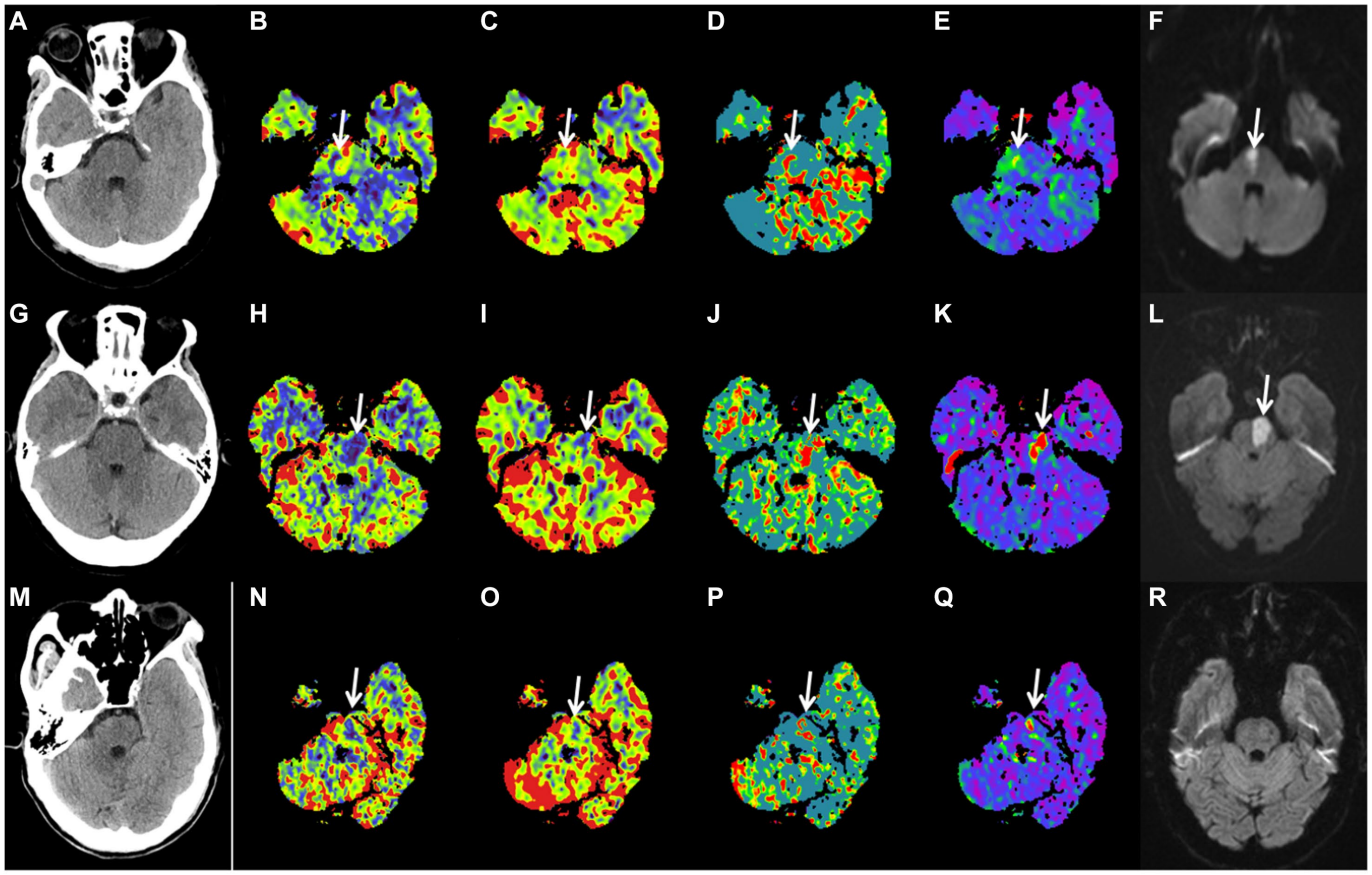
Case examples. Patient examples presented with acute isolated pontine perfusion deficits on computed tomography perfusion and confirmed brainstem infarction by follow-up DWI. No lesion could be detected on noncontrast computed tomography (A,G,M). Patient 1 is a 54-year-old female patient with a baseline right pontine CBF deficit volume of 0.75 mL (B), CBV deficit volume of 0.13 mL (C), MTT deficit volume of 1.55 mL (D), Tmax deficit volume of 2.06 mL (E), and follow-up DWI FIV of 0.91 mL (F). NIHSS score on admission was 5; mRS after 90 d was 0. Patient 2 is a 67-year-old male patient with a baseline left pontine CBF deficit volume of 2.23 mL (H), CBV deficit volume of 0.65 mL (I), MTT deficit volume of 2.35 mL (J), Tmax deficit volume of 1.91 mL (K), and follow-up DWI FIV of 1.96 mL (L). NIHSS score on admission was 6; mRS after 90 d was 3. Patient 3 is a 65-year-old male patient with a baseline left pontine CBF deficit volume of 0.73 mL (N), CBV deficit volume of 0.33 mL (O), MTT deficit volume of 1.08 mL (P), Tmax deficit volume of 1.94 mL (Q), and follow-up DWI negative findings (R). NIHSS score on admission was 6; mRS after 90 d was 0. DWI, diffusion-weighted imaging; CBF, cerebral blood flow; CBV, cerebral blood volume; MTT, mean transit time; and Tmax, time to maximum; FIV, final infarction volume; NIHSS, National Institutes of Health Stroke Scale; mRS, modified Rankin Scale.
